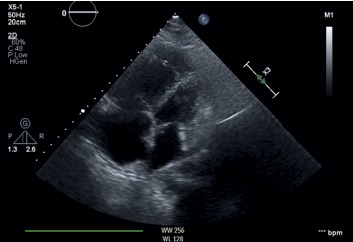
Echodcardioraphy apical 4 chamber view showing right atrial and right ventricular dilation.
